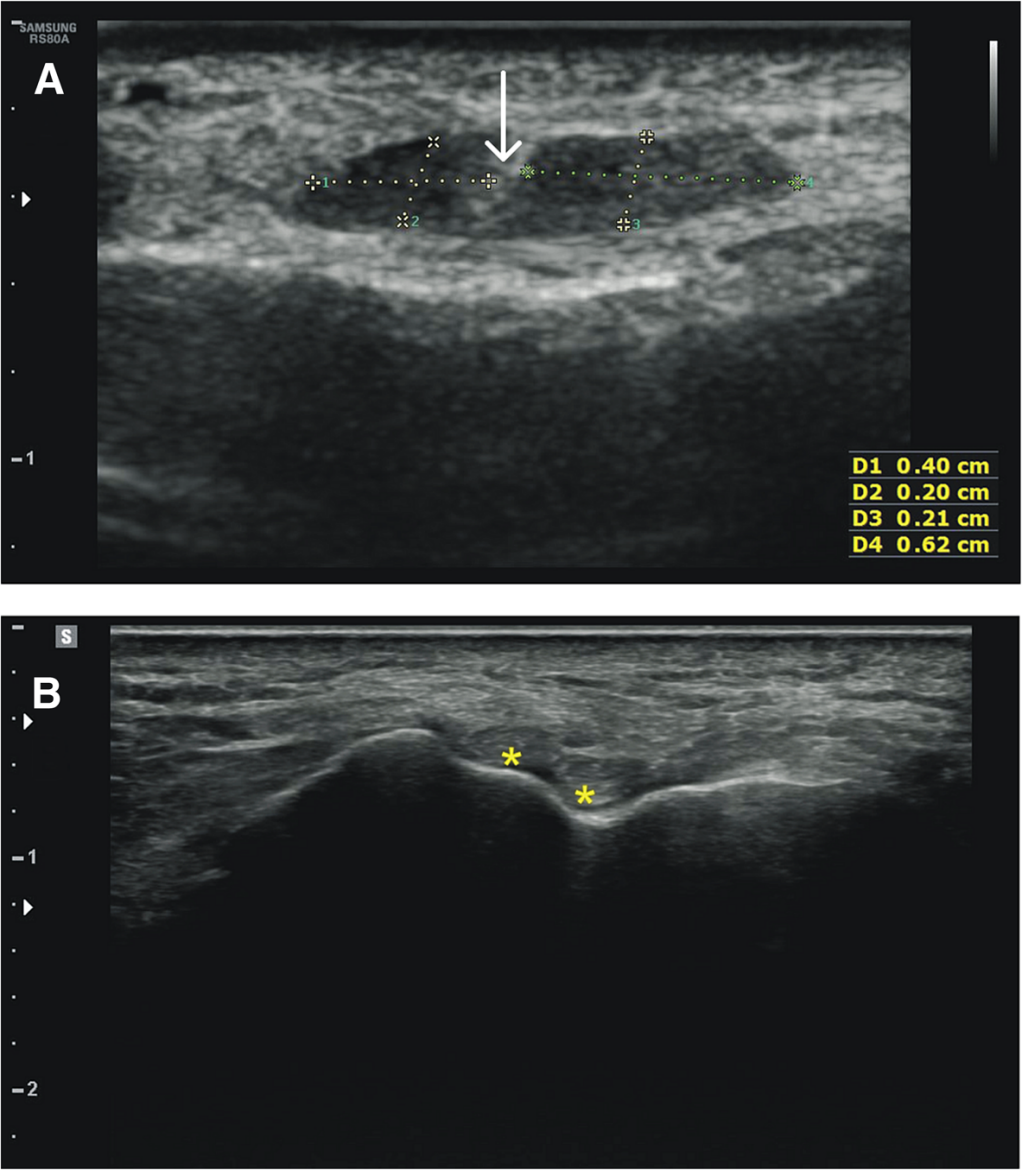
Type VI tibialis anterior tendon. Sonographic view of the cross-section of the tibialis anterior tendon in proximal section (a) and in distal section (b), close to the attachment. In proximal section (a) two components of the tendon are separated by a septa (arrow). In distal section (b) components (each marked with the asterix) are separated and travel in discrete notches on the medial cuneiform bone
